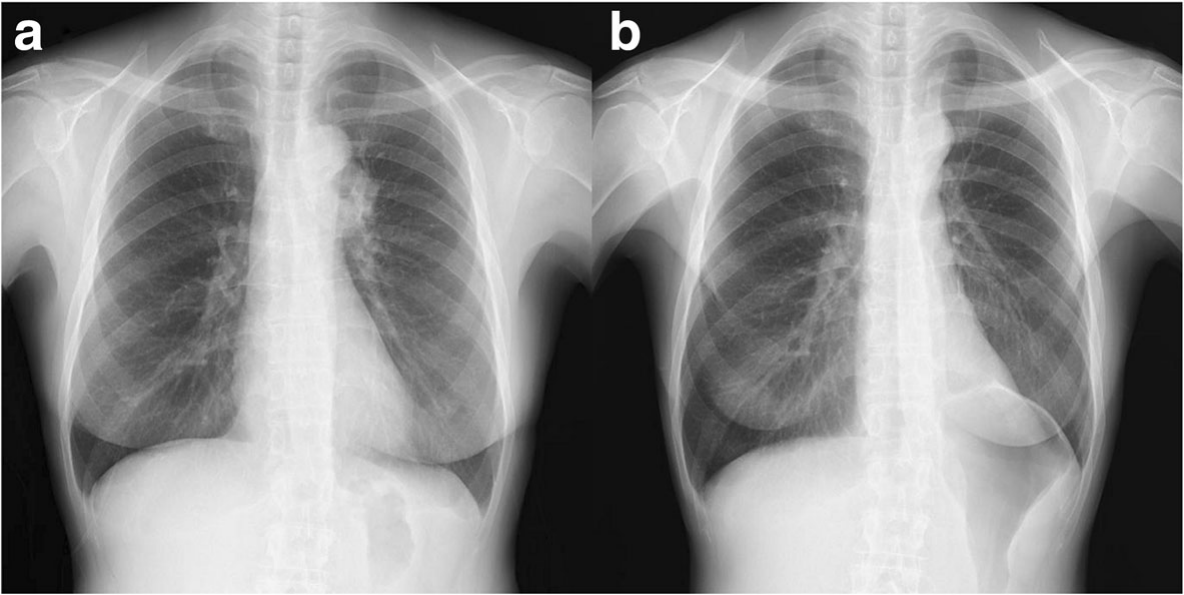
a Preoperative chest radiograph and b postoperative chest radiograph at 1 year showing mild elevation of the left hemidiaphragm
